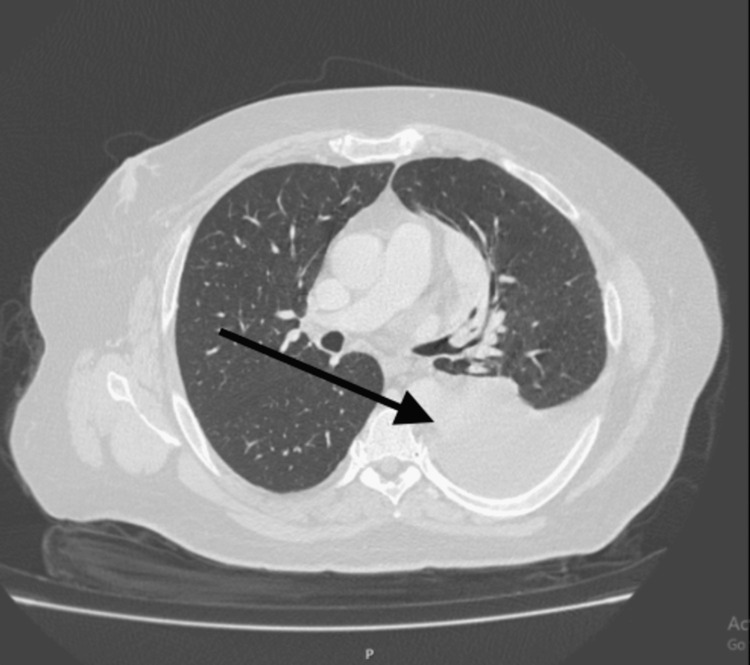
CT scan showing a lung mass with mediastinal involvement and extensive metastatic disease.
